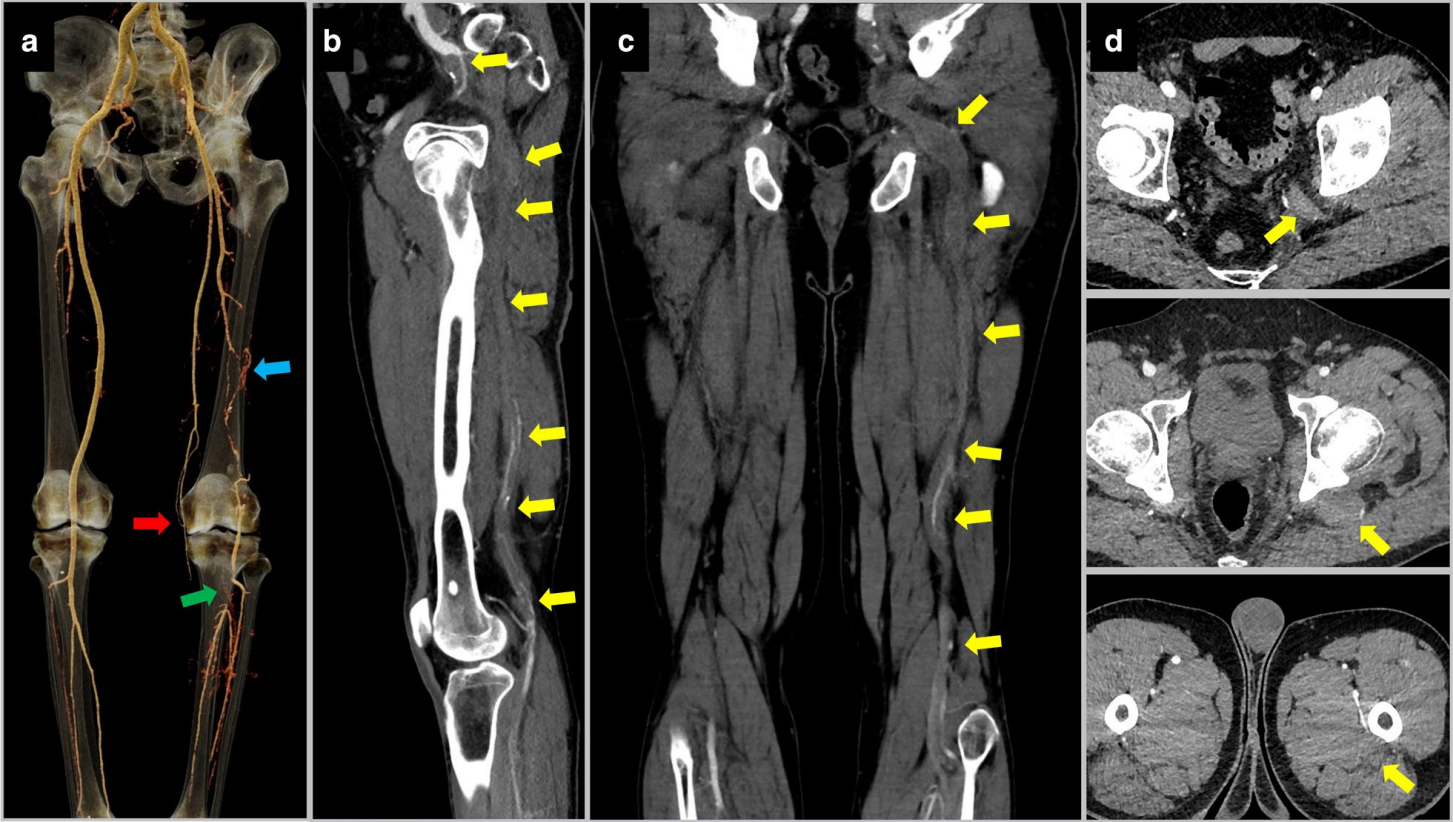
Lack of knowledge (type 3) error. A 55-year-old male patient underwent lower extremity CTA due to signs of peripheral artery disease in the left lower extremity. The radiology resident who examined the CTA for the preliminary results saw that the left superficial femoral artery was of thin caliber and ended at the knee level. He thought that it was chronically occluded (red arrow, a). He also thought that collaterals between the left deep femoral artery and popliteal artery (blue arrow, a) had developed secondary to the thrombi in the left popliteal artery (green arrow, a). When the radiologist referred the CTA to the attending physician, he learned that the patient’s left internal iliac artery continued as the popliteal artery, which is a variation called persistent sciatic artery. The persistent sciatic artery of the patient was thrombosed throughout its course (yellow arrows, b, c, d). The resident could not recognize this variation and the pathology due to the lack of knowledge. Although this is not a common variation that should be known by every radiologist, in this case, failure to recognize the thrombosed persistent sciatic artery could have adversely affected the possible interventional treatment plan
